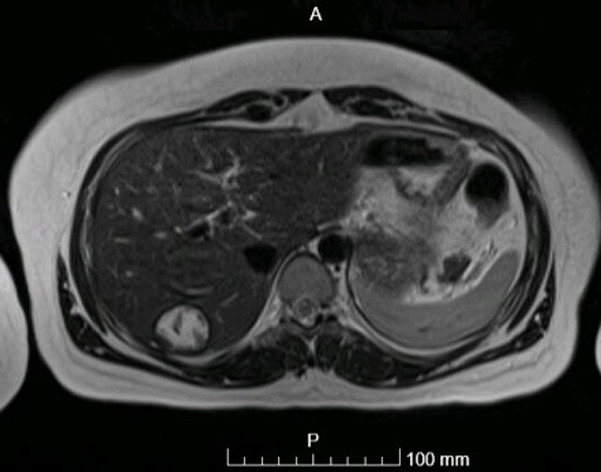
MRI abdomen showing the liver mass with a segment 6/7 cyst with T2 dark rim and dark intra-cystic areas corresponding to calcifications by CT (most probably a calcified hydatic cyst)
